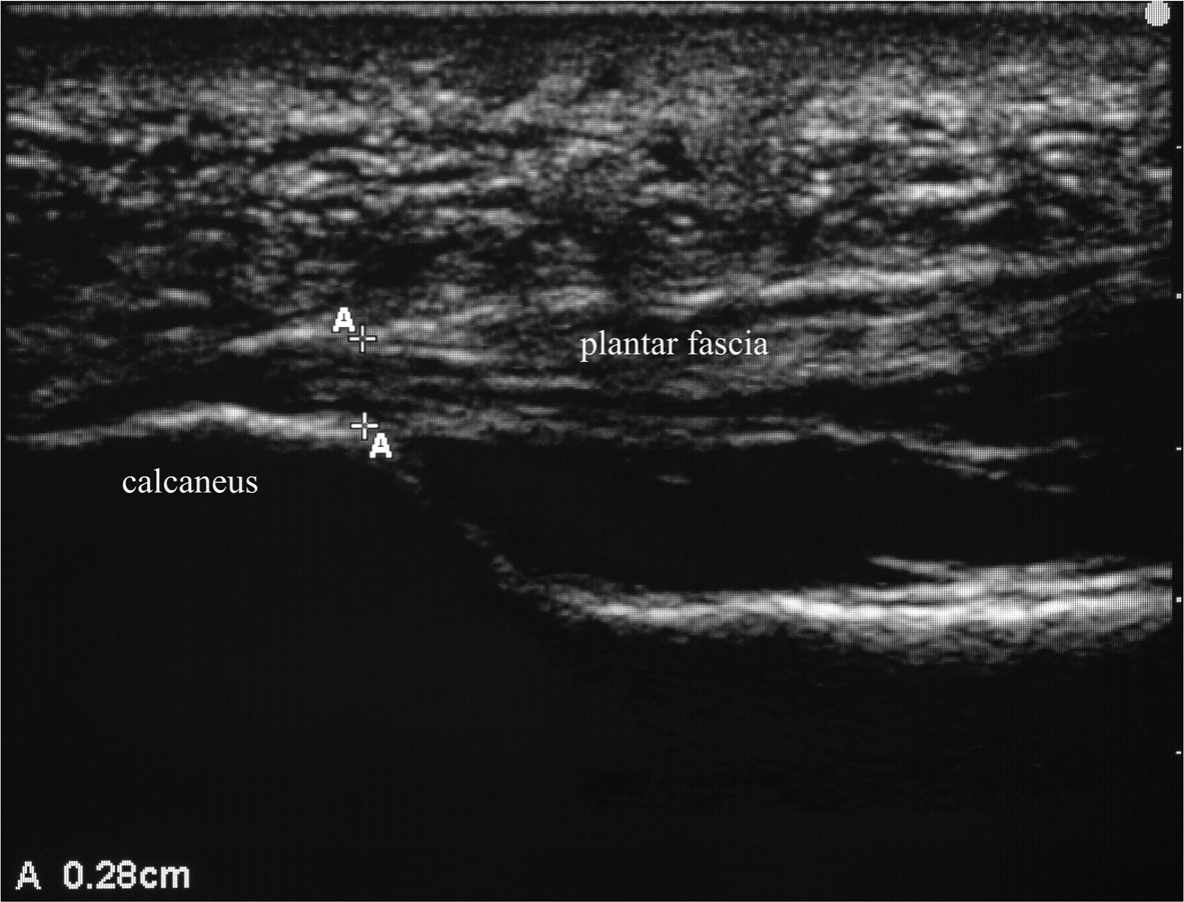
Longitudinal sonogram of the plantar fascia with the thickness being measured at the anterior margin of the calcaneus
